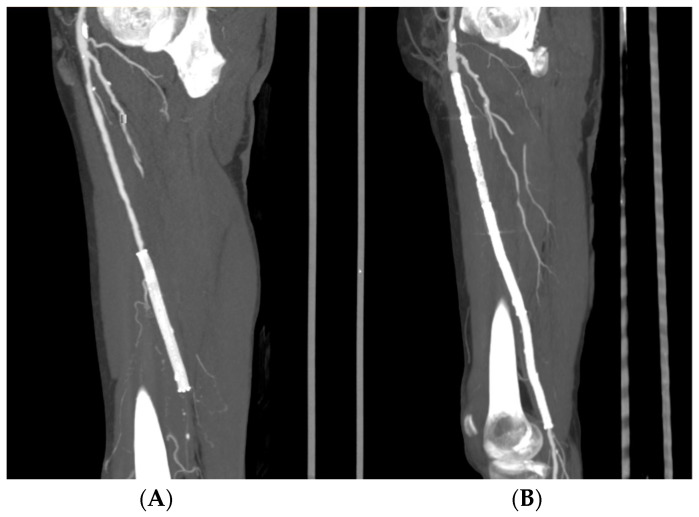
Preoperative CT MPR showing multilevel peripheral arterial disease compared to a common superficial artery, an in stent occlusion, and a distal superficial femoral artery occlusion (A). Postoperative CT MPR showing the outcome after common femoral artery patch angioplasty, stent recanalization, and superficial femoral artery stenting (B).
